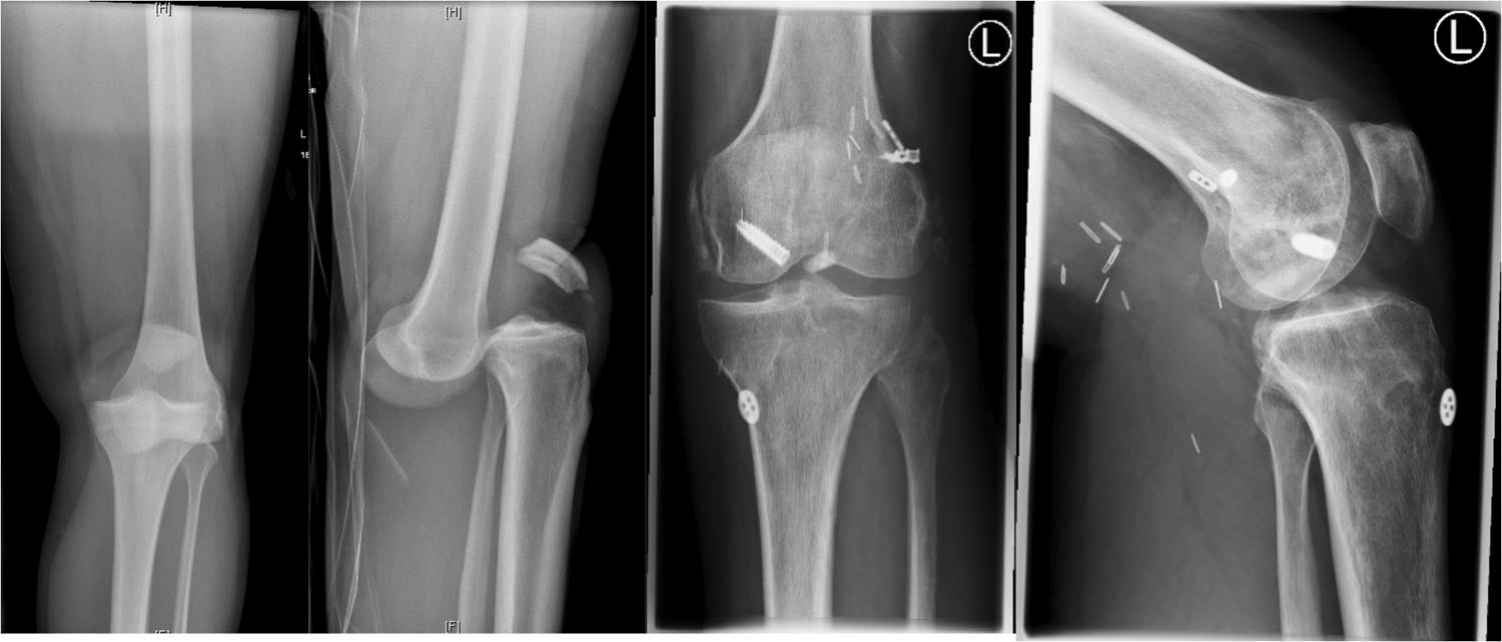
Anterior–posterior and lateral radiographs of a posterior dislocation of the knee (Grade-A) and the joint after reduction, vain graft of the popliteal artery and surgical ligament repair of the posterior cruciate ligament (PCL), the anterior cruciate ligament (ACL), and the lateral collateral ligament complex (LCL)
